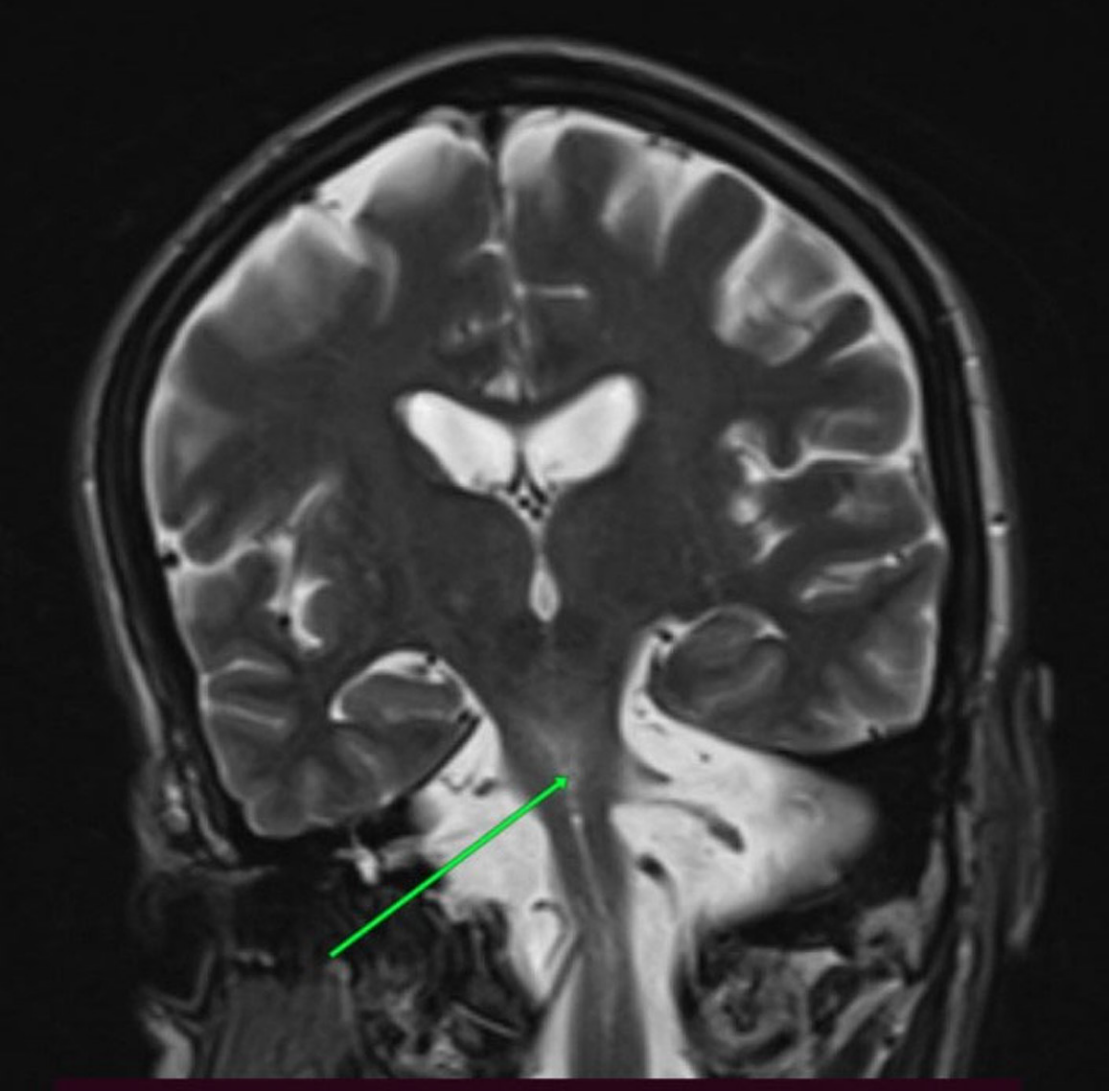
Pontine degeneration on T2‐weighted MRI(green arrow).
